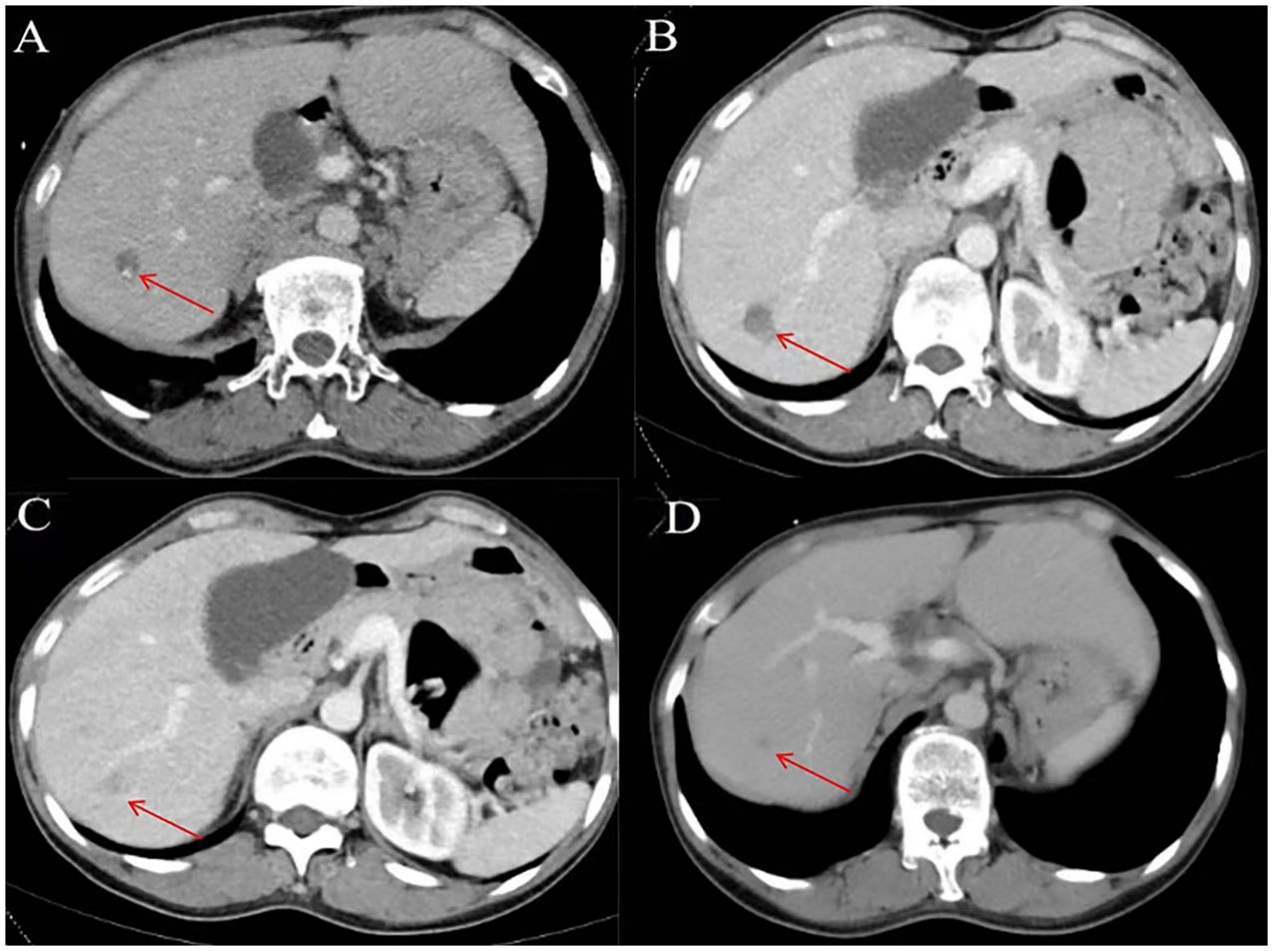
Representative images depicting lesions before and 1, 6, and 12 months after microwave ablation (MWA) in a 54-year-old female with hepatoalveolar echinococcosis (HAE). (A) Enhanced computed tomography (CT) performed before ablation; (B) Enhanced computed tomography (CT) 1 month after ablation; (C) Enhanced computed tomography (CT) 6 months after ablation; (D) Enhanced computed tomography (CT) 12 months after ablation. The red arrow indicates the HAE lesion.
